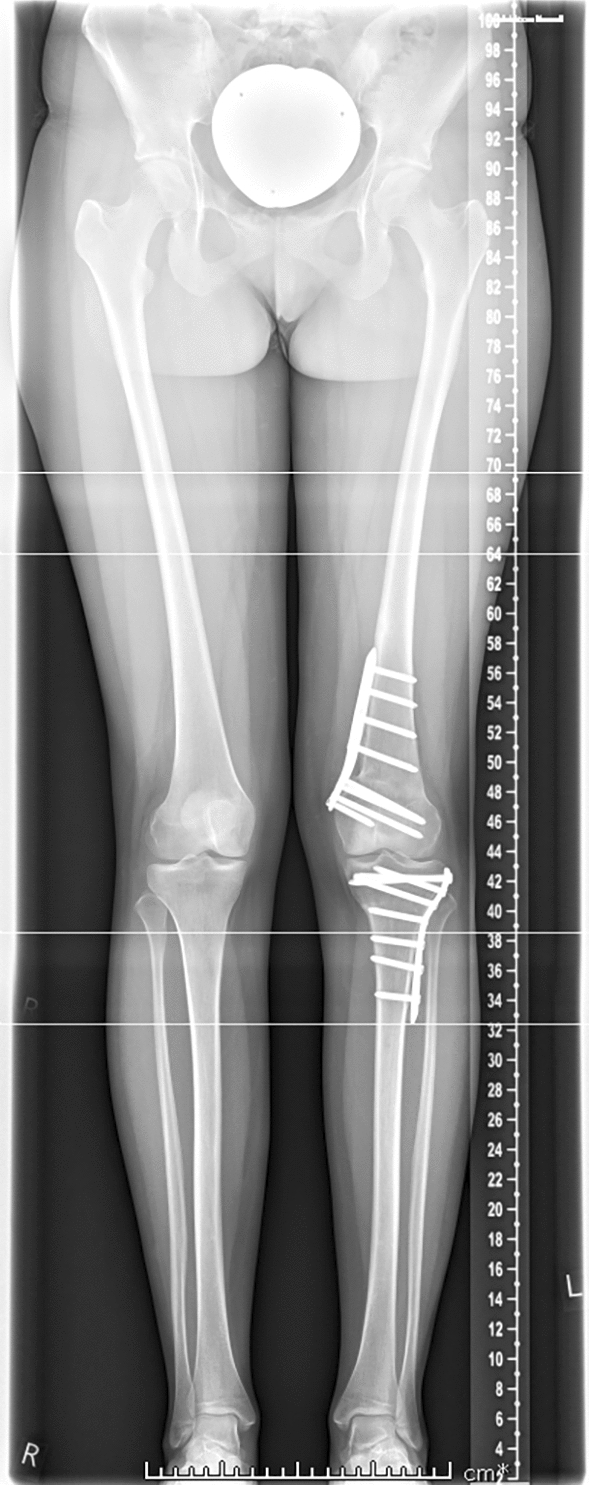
Post-operative x-rays long leg view of a 17 year-old female 10 months after double level osteotomy (femoral external and tibial internal rotational osteotomy) and osteosynthesis, bony consolidation
